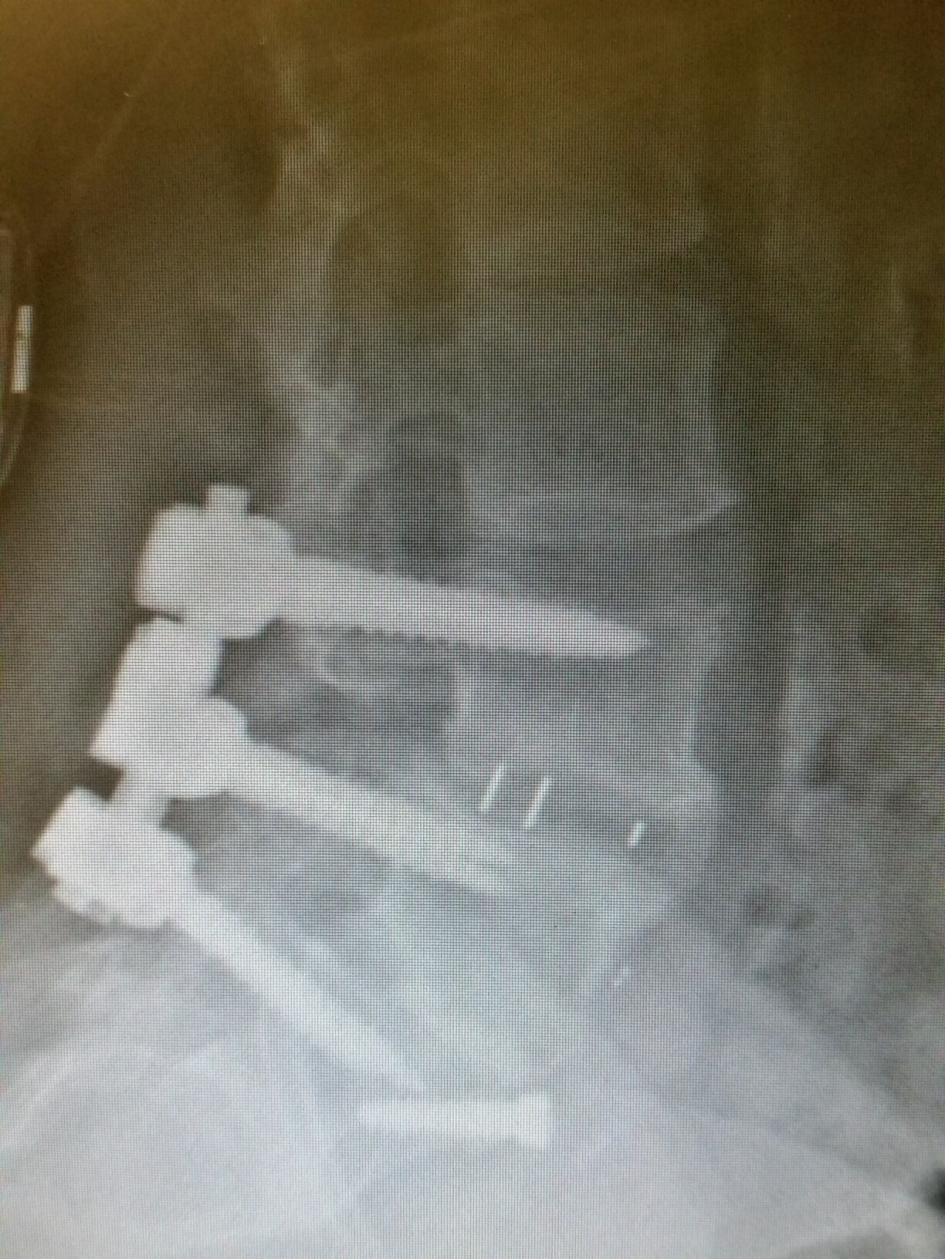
Lateral X-ray appears to show the graft in place; however, L4-5 is not fused.
